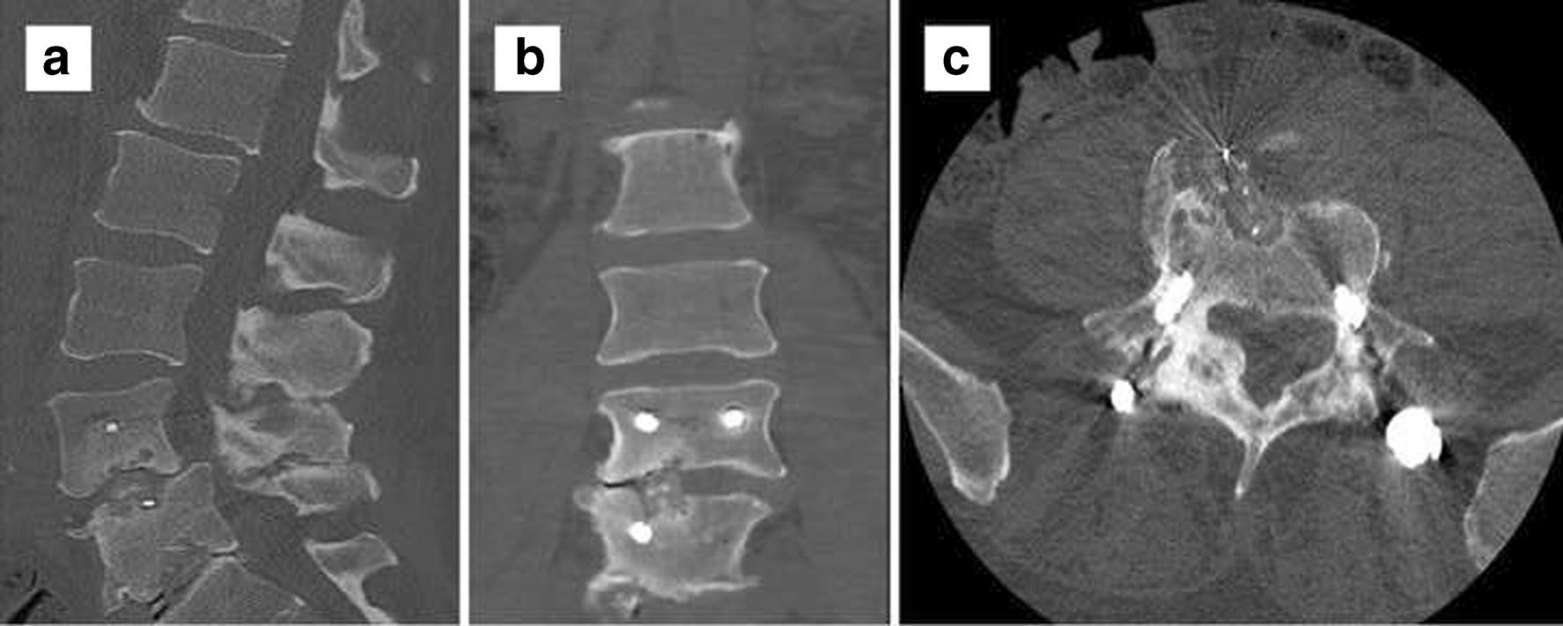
Sagittal, coronal, and axial CT images show migration of the fusion cage through the superior endplate of more than 3 mm resulting in loss of height restoration and subsidence
